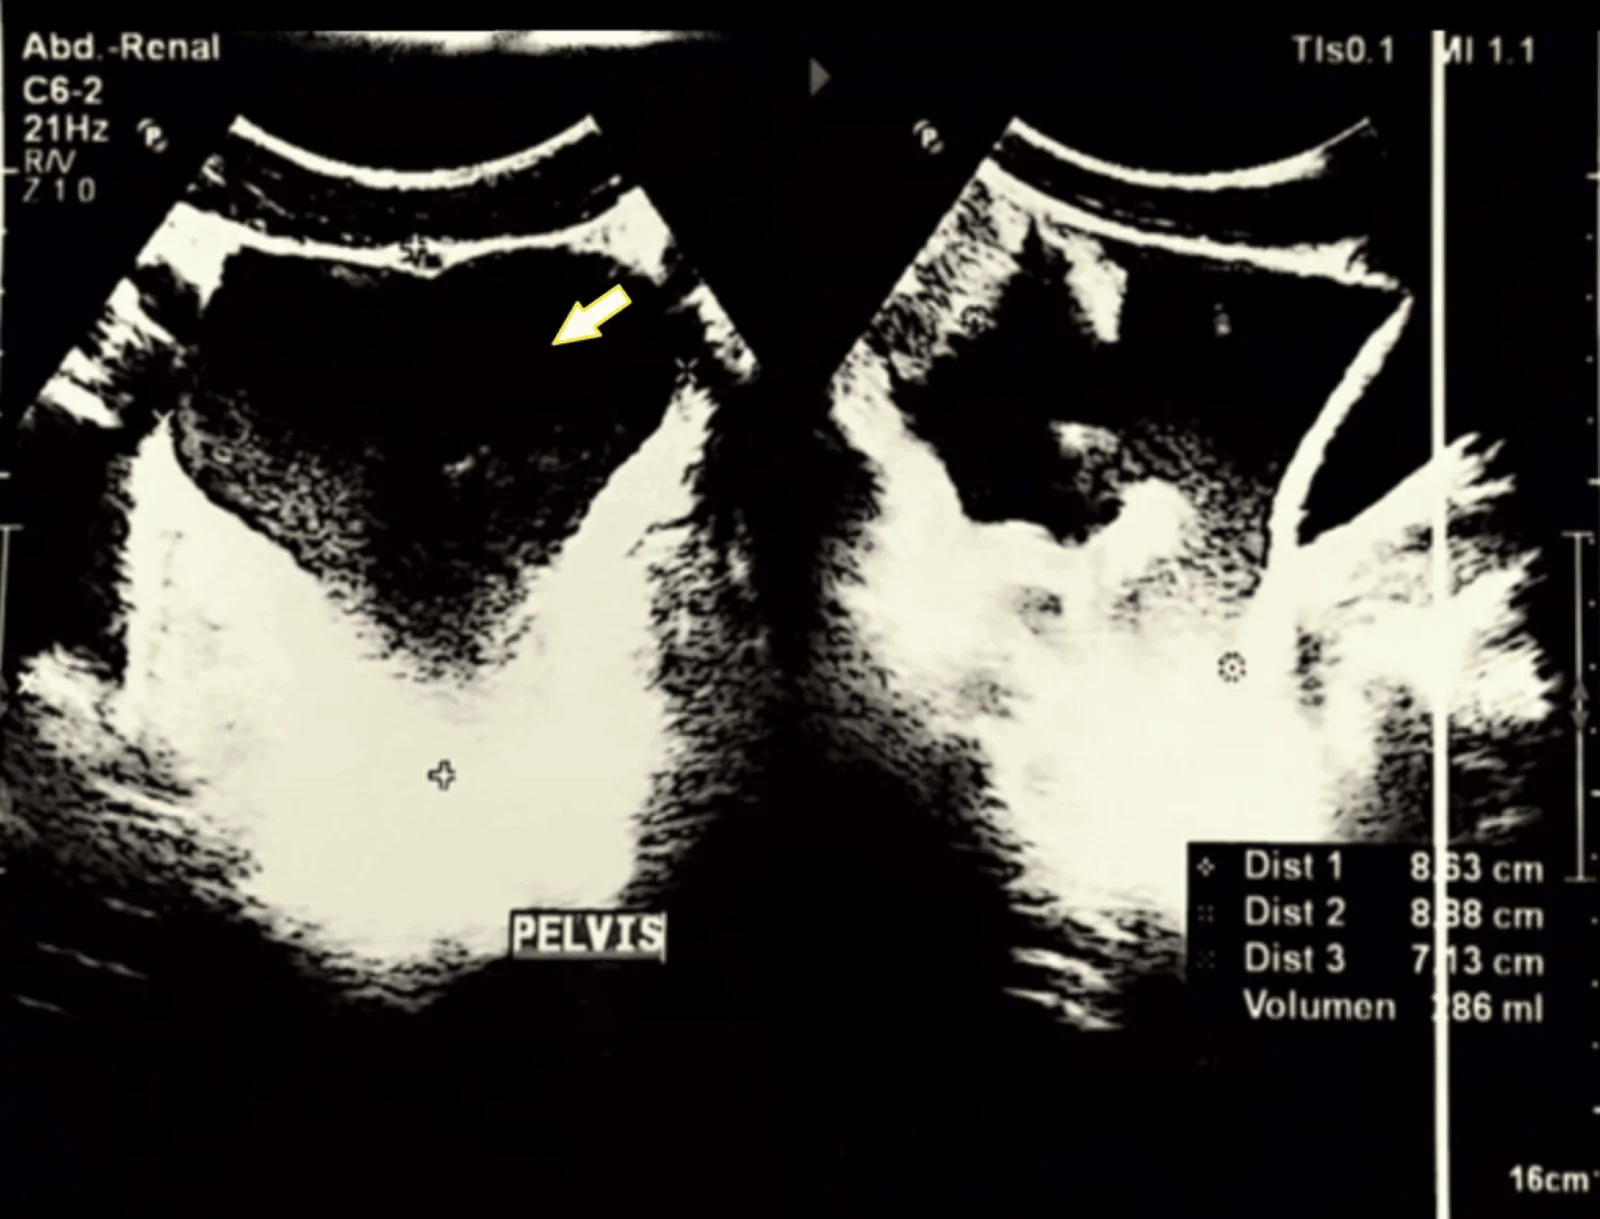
Preoperative pelvic ultrasonography demonstrating free intraperitoneal fluid within the pelvic cavity. The arrow indicates the free fluid collection.
